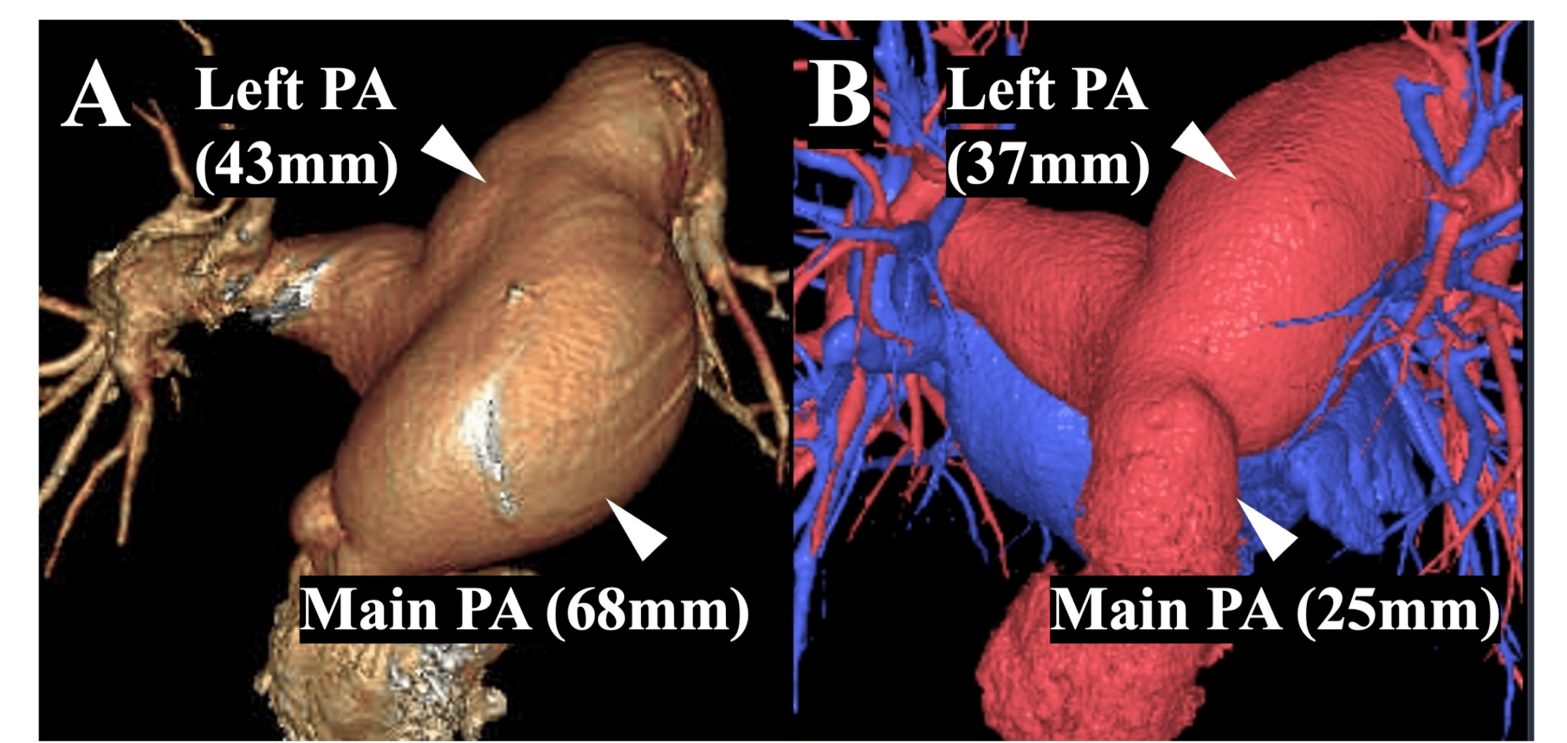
Comparison of 3D CT (A, preoperative; B, two-year postoperative) Preoperative CT showed a massively dilated main PA (68 mm) and a dilated proximal left PA (43 mm) (A). A two-year postoperative CT showed a stable main PA diameter of 25 mm, an unchanged right PA, a reduced caliber of the left PA compared with the preoperative 43 mm, and no graft-related complications (B). PA: pulmonary artery
